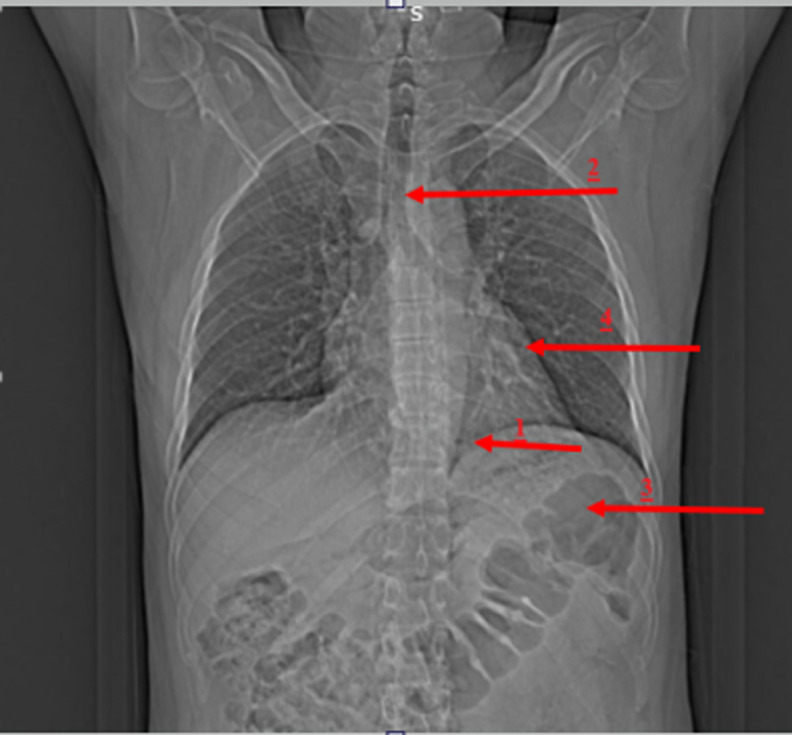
computed tomography showing invasion of abdominal contents into thoracic cavity due to herniation
